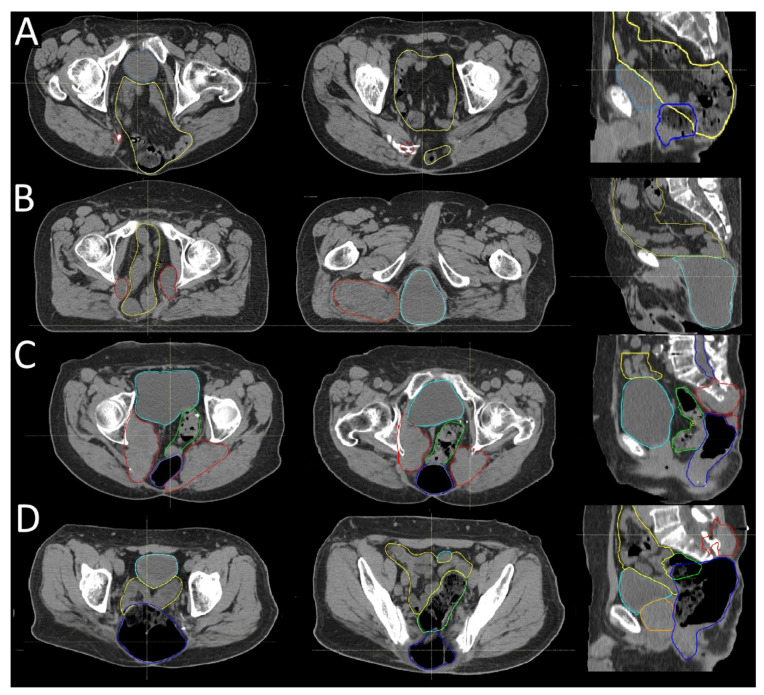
Anatomical changes following sacrectomy. Representative axial and sagittal CT slices from four sacral chordoma patients after surgery. In image (A), the small bowel (yellow) is visible. In image (B), the rectum has been surgically removed, and the bladder (light blue) now occupies that anatomical region. Images (C,D) show a typically distended rectum (dark blue) located close to the skin surface. Recurrent chordoma lesions are marked in red. Other depicted structures are the prostate (orange) and sigmoid collon (green).
